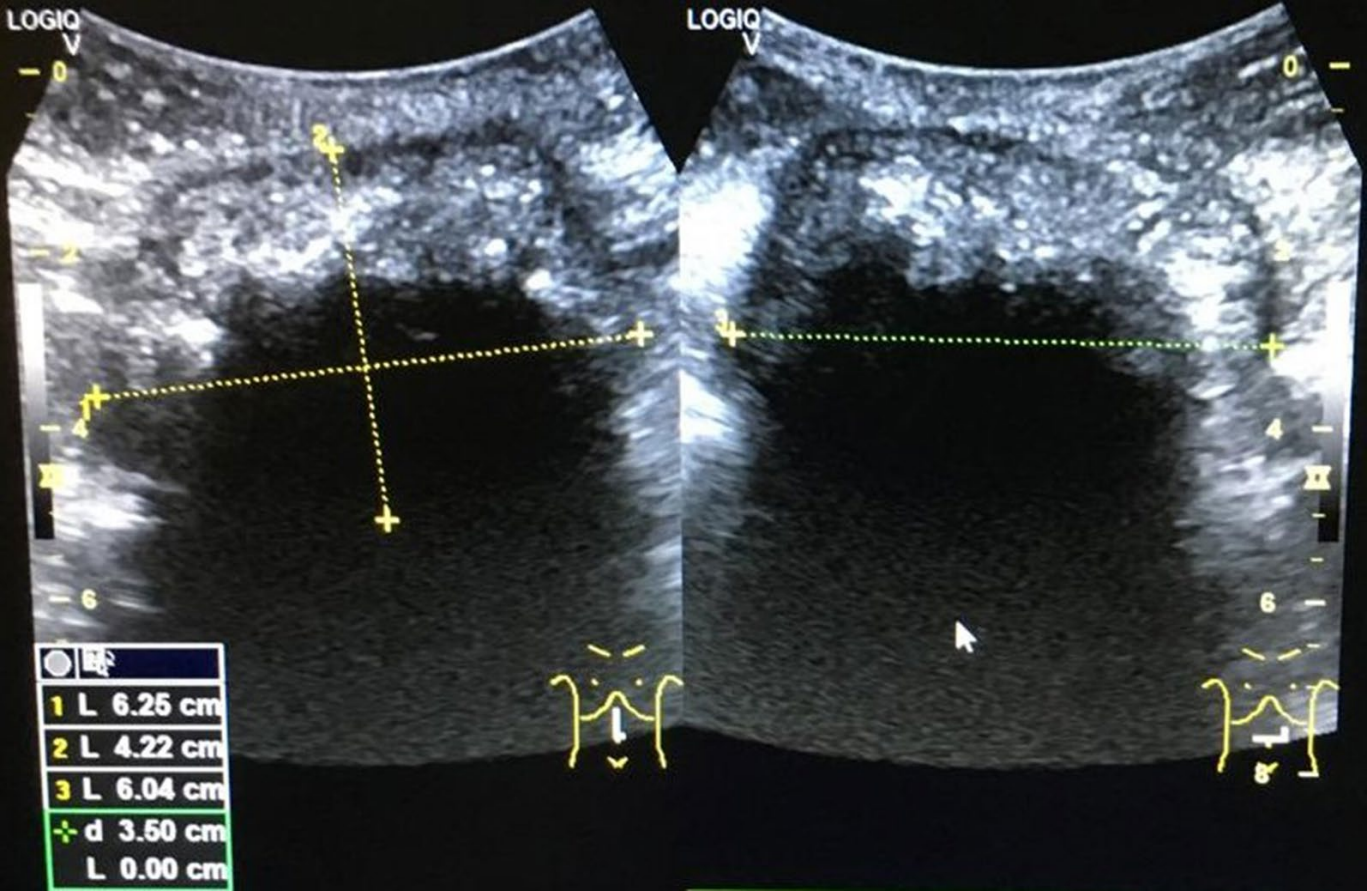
Ultrasound scan showing a well-circumscribed intraabdominal lesion with a complex and irregular internal echo pattern. Strong echoes and posterior acoustic shadowing indicate gas bubbles. Appearances are suspicious of an intraabdominal abscess
